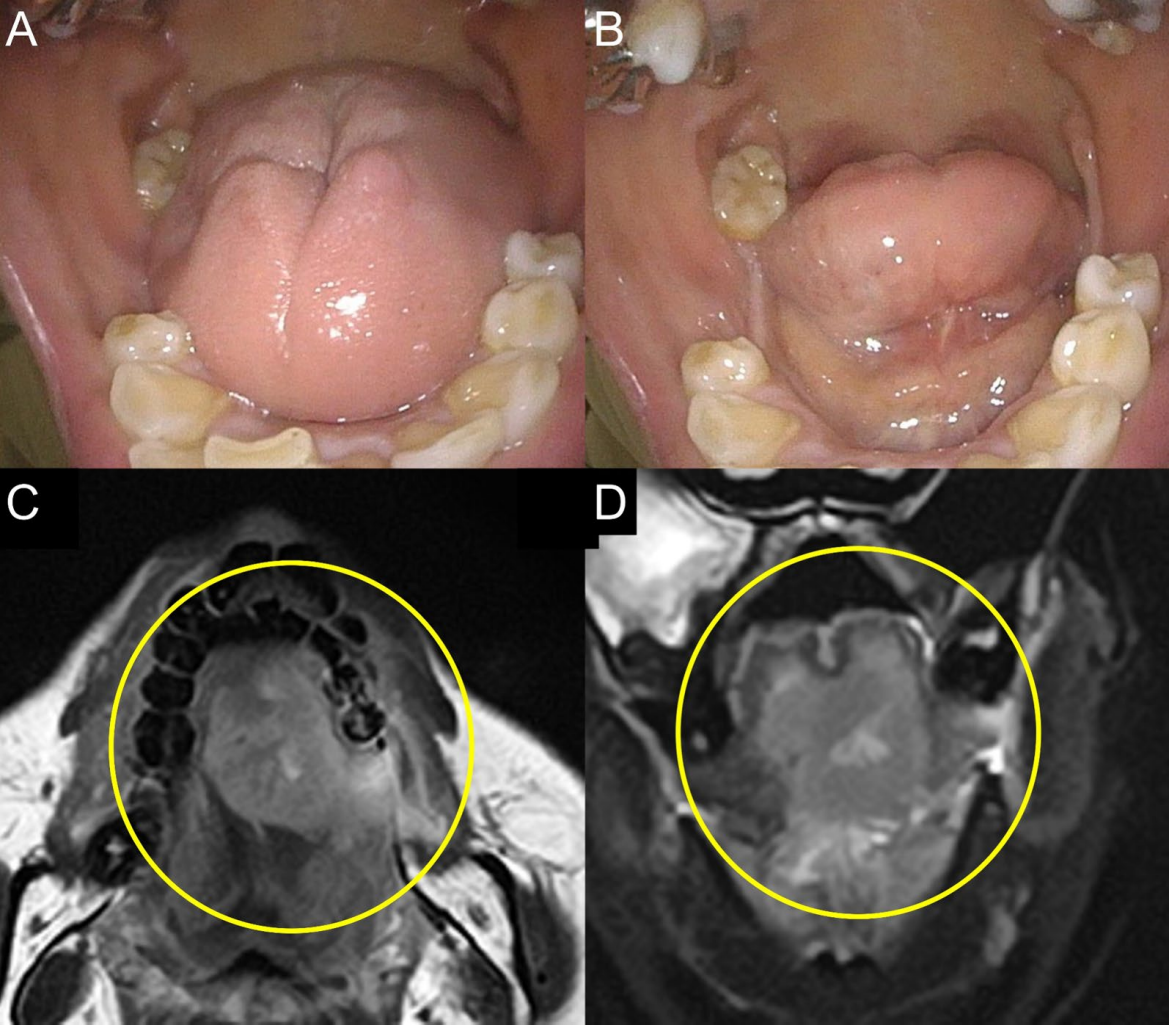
A, B The tongue showed no abnormal appearance. C, D Head MRI findings revealed tongue metastasis
